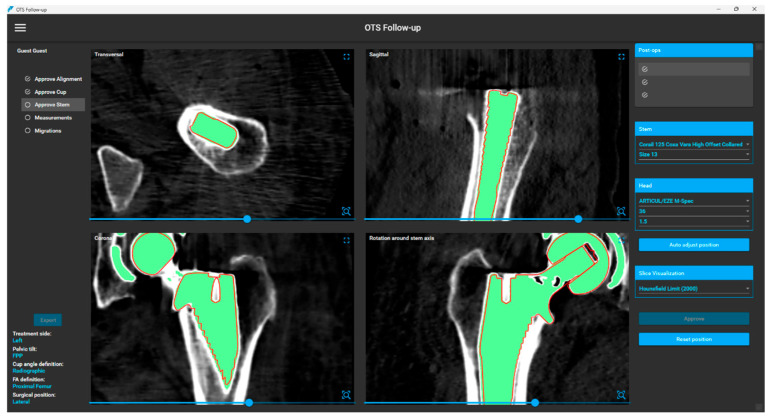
Overview of the AI-based CT-RSA tool for stem implant detection. The segmentation of the implant is visually represented in green, while the contour of the implant’s CAD model, following subsequent alignment, is depicted in red.
